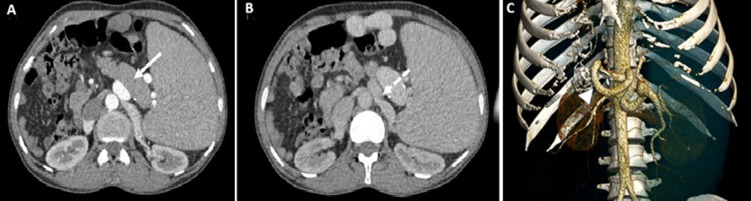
computed tomography (CT) scan and 3D reconstruction demonstrating nutcracker syndrome: A,B) axial CT views and 3D reconstruction; C) an enlarged and serpentine splenic artery (solid arrow), measuring 13mm at its most dilated point; the artery compresses the left renal vein (dotted arrow) against the abdominal aorta, producing a 'beak sign; the hilar-to-compression diameter ratio is 7
